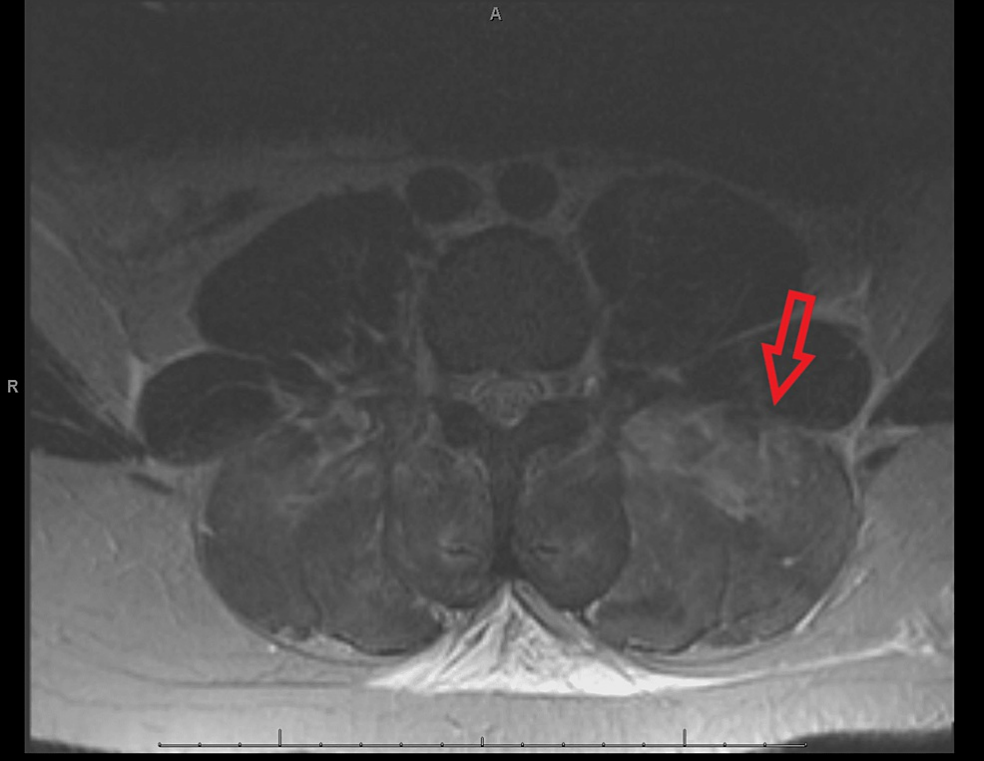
T2-weighted MR sequence demonstrating hyperintense homogenous signal in bilateral paravertebral compartments, left worse than right.
